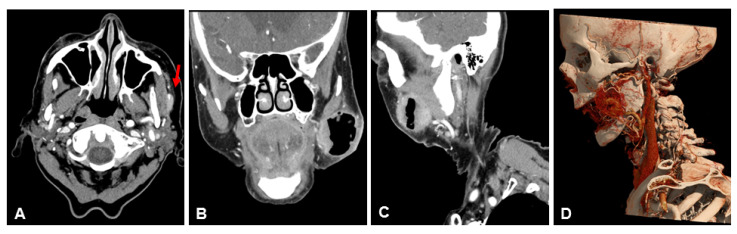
Example of a 93-year-old female patient with a squamous cell carcinoma of the left cheek. UHR-CT enables an accurate identification of arterial supply and venous drainage of the neoplasm, which is clearly depicted with 3D-reconstruction. It is possible to precisely differentiate between perineural tumor spread (red arrow) and adjacent muscle. (A) axial 3 mm reconstruction UHR-CT, (B) coronal 3 mm reconstruction UHR-CT, (C) sagittal 3 mm reconstruction UHR-CT, (D) best possible 3D-reconstruction NR-CT.
